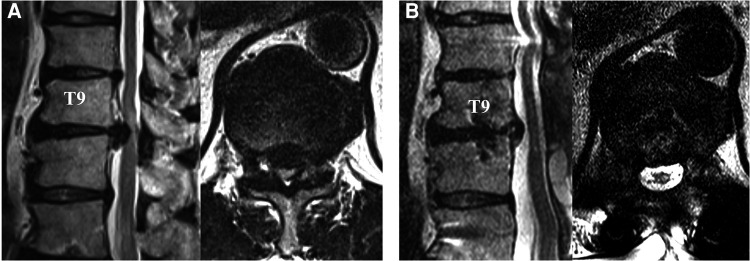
Pre- (A) and postoperative MRI (B) of the patient, a 45-year-old male, with ossification of the posterior longitudinal ligament (OPLL) at T9–10. (A) The spinal cord is severely compressed from anteriorly by OPLL at T9–10 with significant T2 high signal intensity. (B) After our modified Ohtsuka procedure, the spinal cord was completely decompressed, and cerebrospinal fluid was confirmed at the ventral side of the spinal cord.
